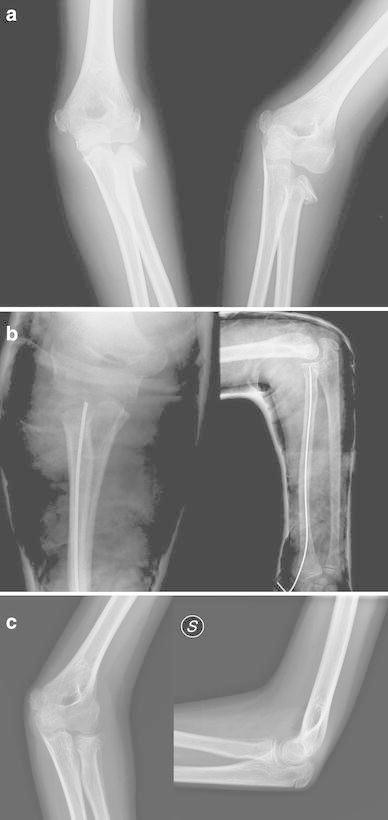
a Radial neck fracture Judet type III. b Osteosynthesis with ESIN, postoperative X-rays. c X-rays at 3 months, after nail removal, showing an excellent alignment
